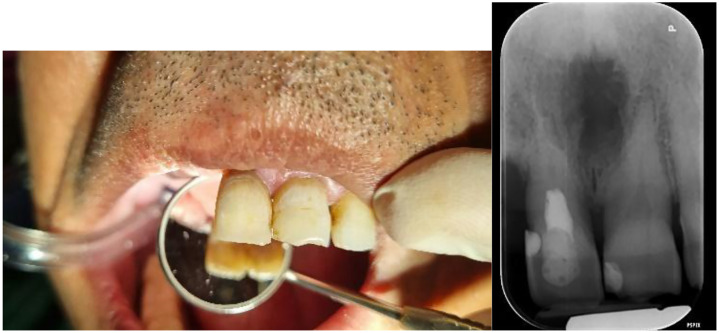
Periapical radiography and photography after root canal therapy and tooth restoration.
